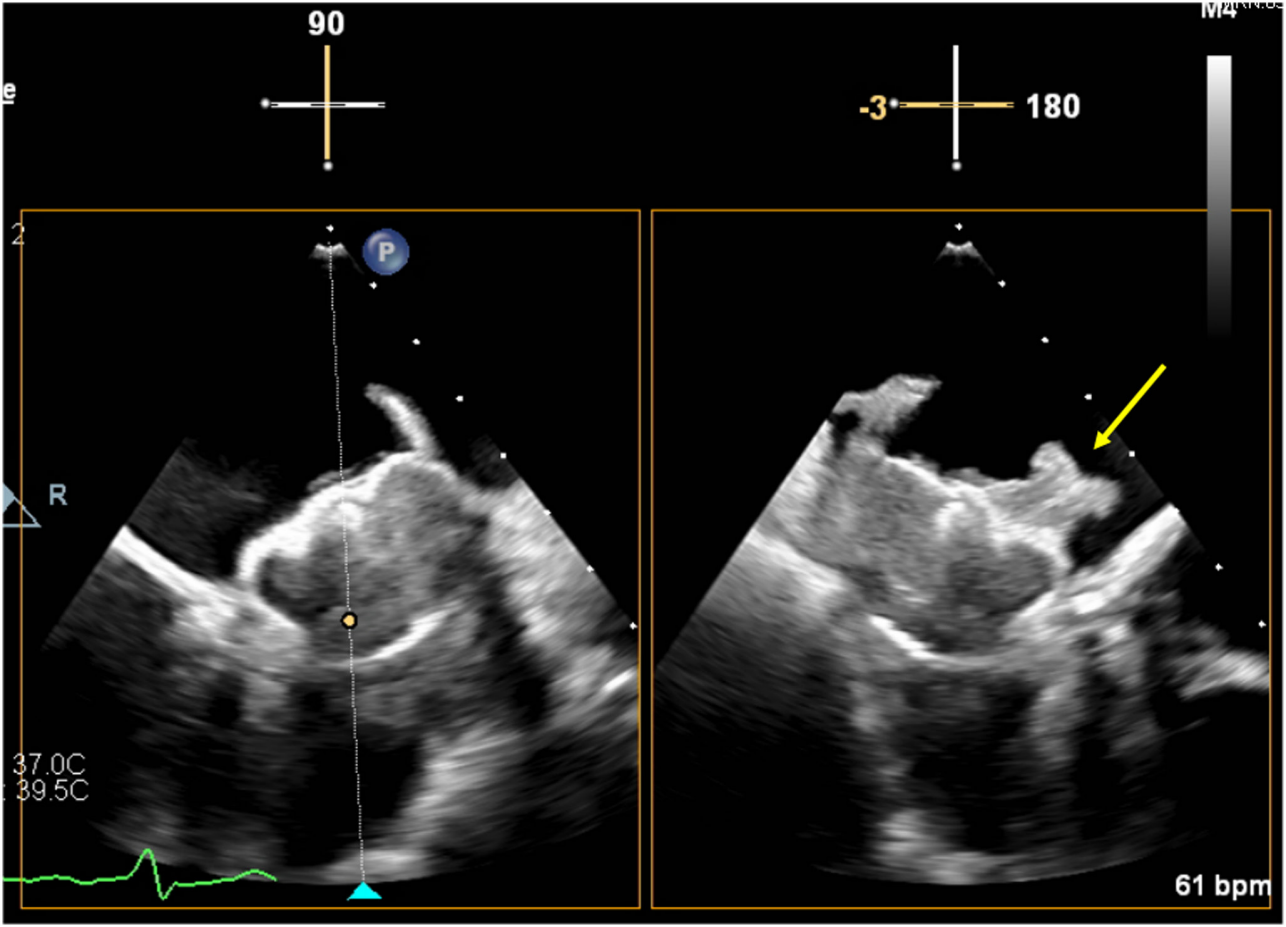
Transesophageal echocardiography image revealing a pedunculated and mobile device‐related thrombosis (yellow arrow) at the time of ischemic stroke diagnosis.
